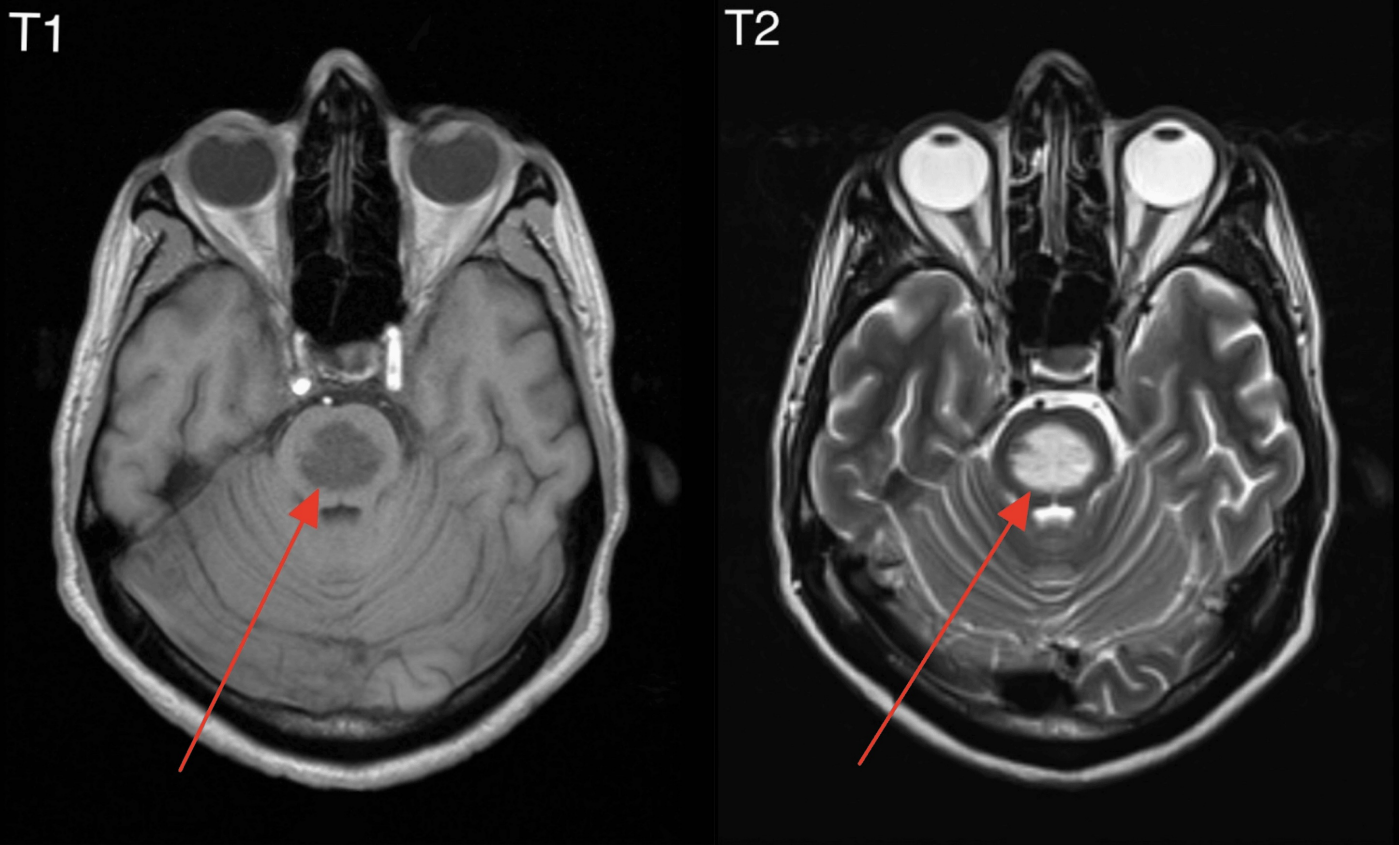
MRI of the brain in T1 and T2 sequences showing a well-demarcated, rounded signal intensity located in the central pons, sparing the peripheries, specifically the ventrolateral longitudinal fibers and corticospinal tracts.
